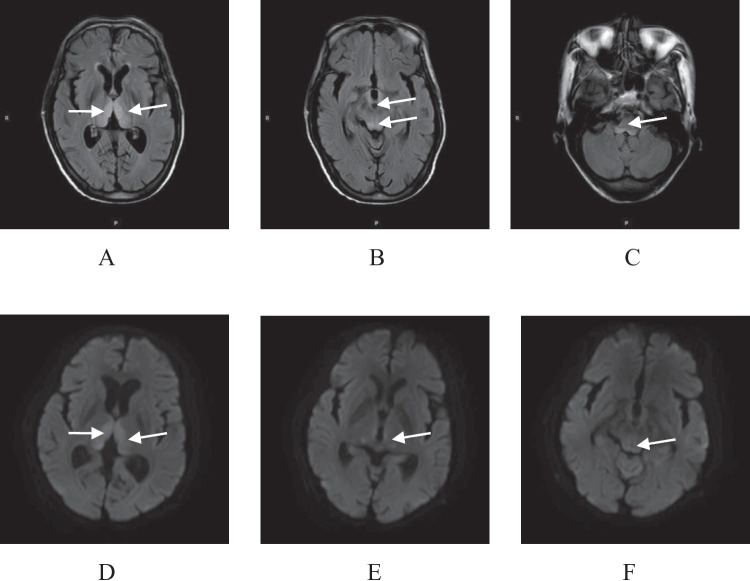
Fig. 1 MRI of the brain. T2-FLAIR imaging demonstrated symmetric hyperintensities (arrows) in the bilateral medial dorsal thalamus (A), periventricular region around the third ventricle and tectum (B), and dorsal medulla oblongata (C). DWI demonstrated symmetric hyperintensities (arrows) in the bilateral medial dorsal thalamus (D), geniculate body (E), and the region around the periaqueductal grey matter (F)
